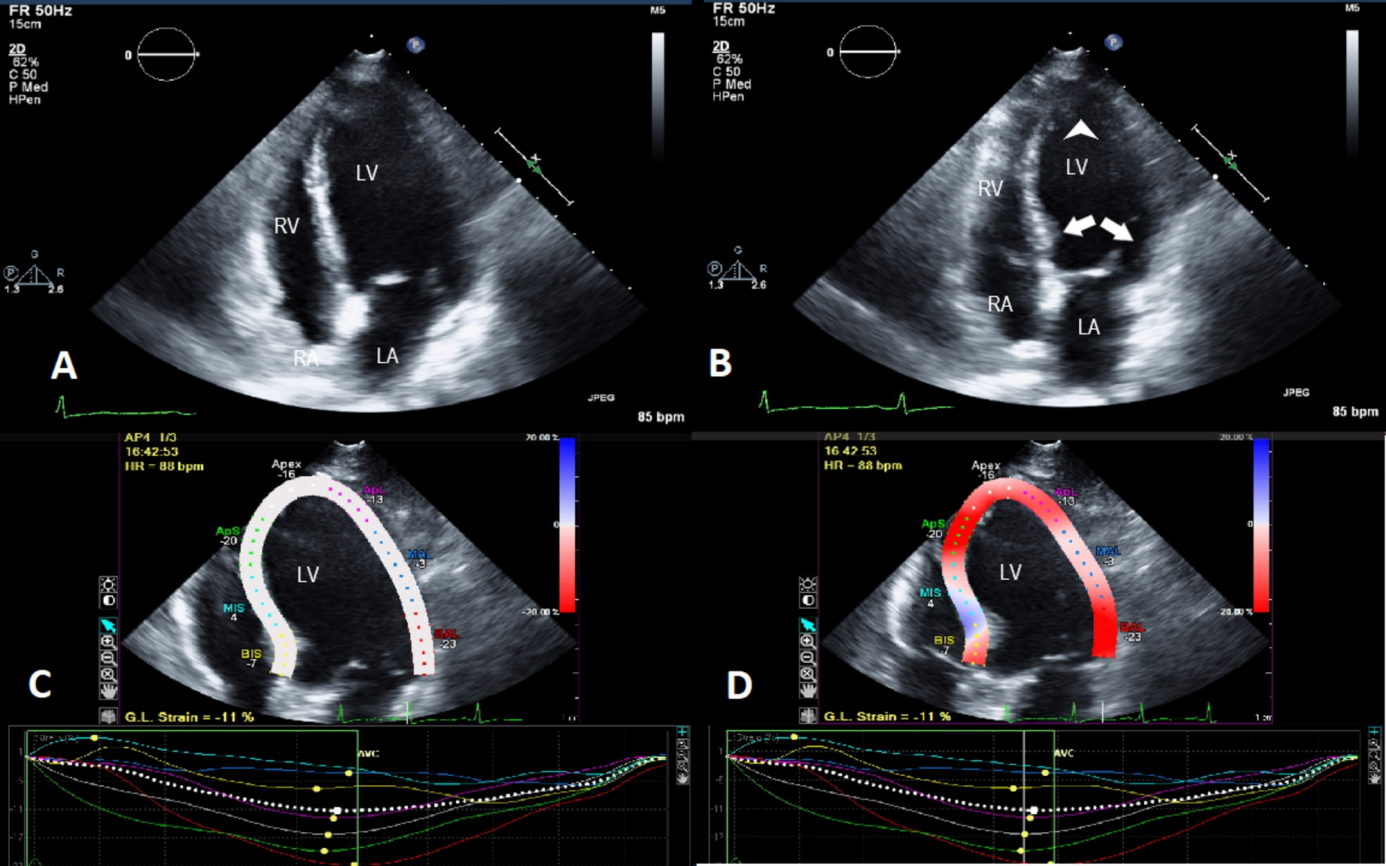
Transthoracic echocardiogram. Panel A represents the four chamber view in end diastole showing global dilatation of left ventricle. Panel B represents four chamber view in end systole demonstrating akinetic left ventricle with sparing of the apical (arrowhead) and basal (arrows) segments. Panel C represents left ventricle wall motion in end diastole and Panel D represents left ventricle wall motion in end systole demonstrating contractility in the basal and apical area with akinesia in the other segments. (LV: Left ventricle, RV: Right ventricle, LA: Left atrium, RA: Right atrium)
